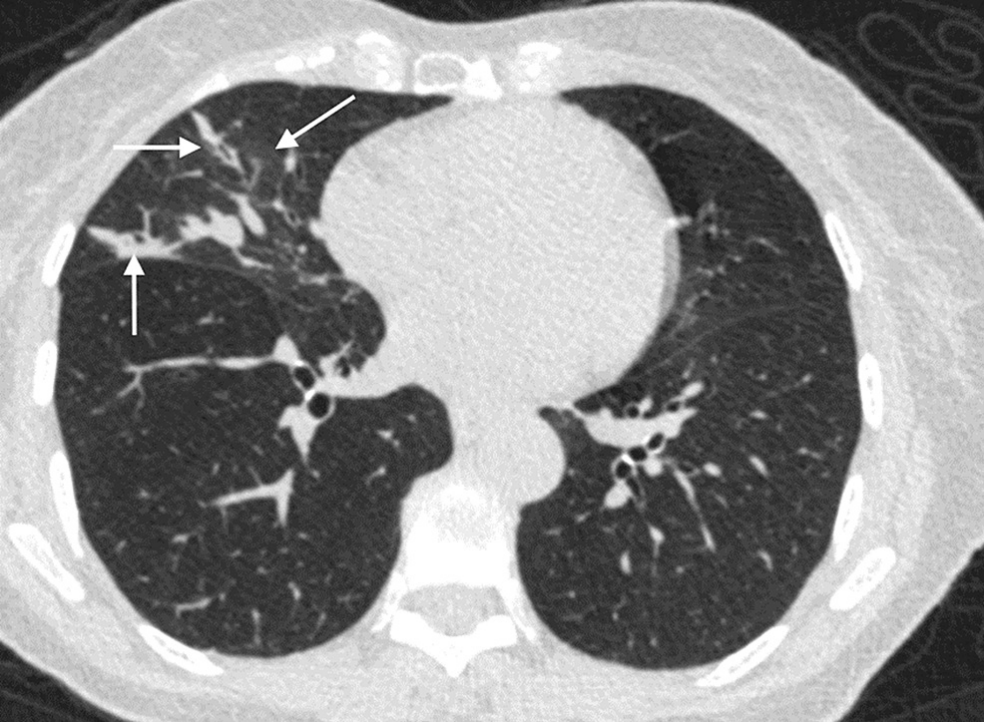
High-resolution CT image through the mid-chest demonstrating ground-glass opacities (oblique arrow), smooth bronchial wall thickening (horizontal arrow), and atelectasis (vertical arrow) within the middle lobe of the right lung.
